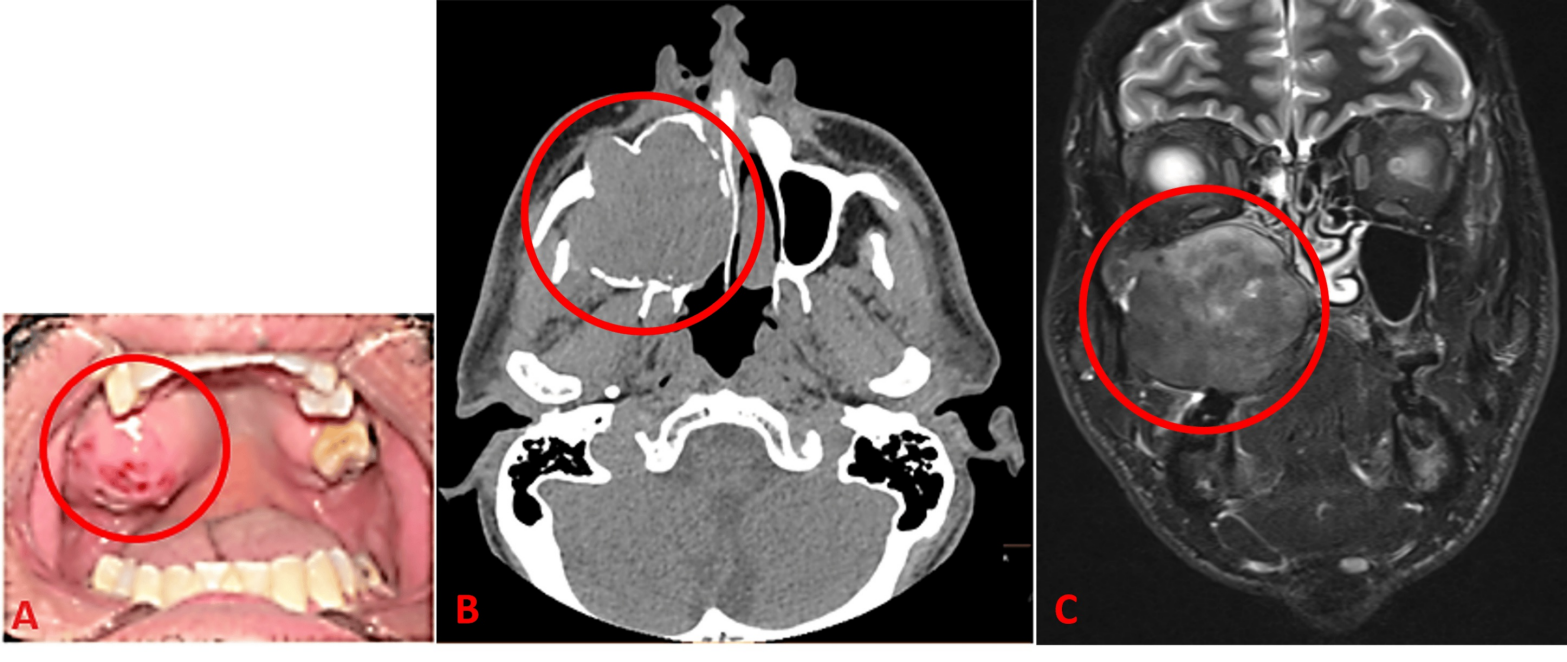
Images of the mass The patient’s oral cavity and maxillary mass (A; mass in red circle). CT (B) and MRI (C) images showing a mass with bone invasion of the maxilla and walls of the maxillary sinus (red circles).
